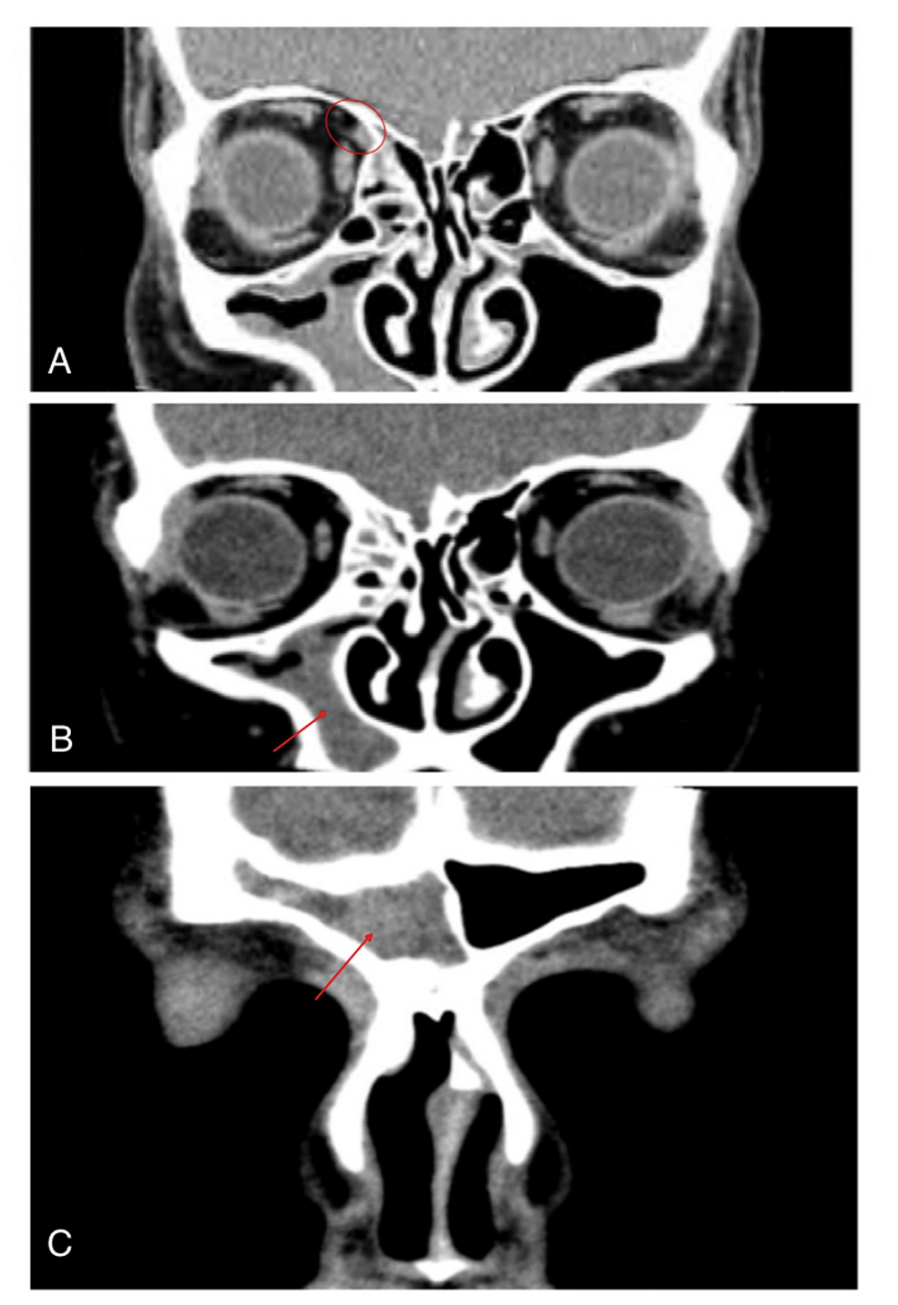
Coronal contrast-enhanced CT scan of orbits and paranasal sinuses showing mild swelling and inflammation of the trochlea of the right eye (A), as well as right-sided maxillary (B) and frontal (C) sinusitis
